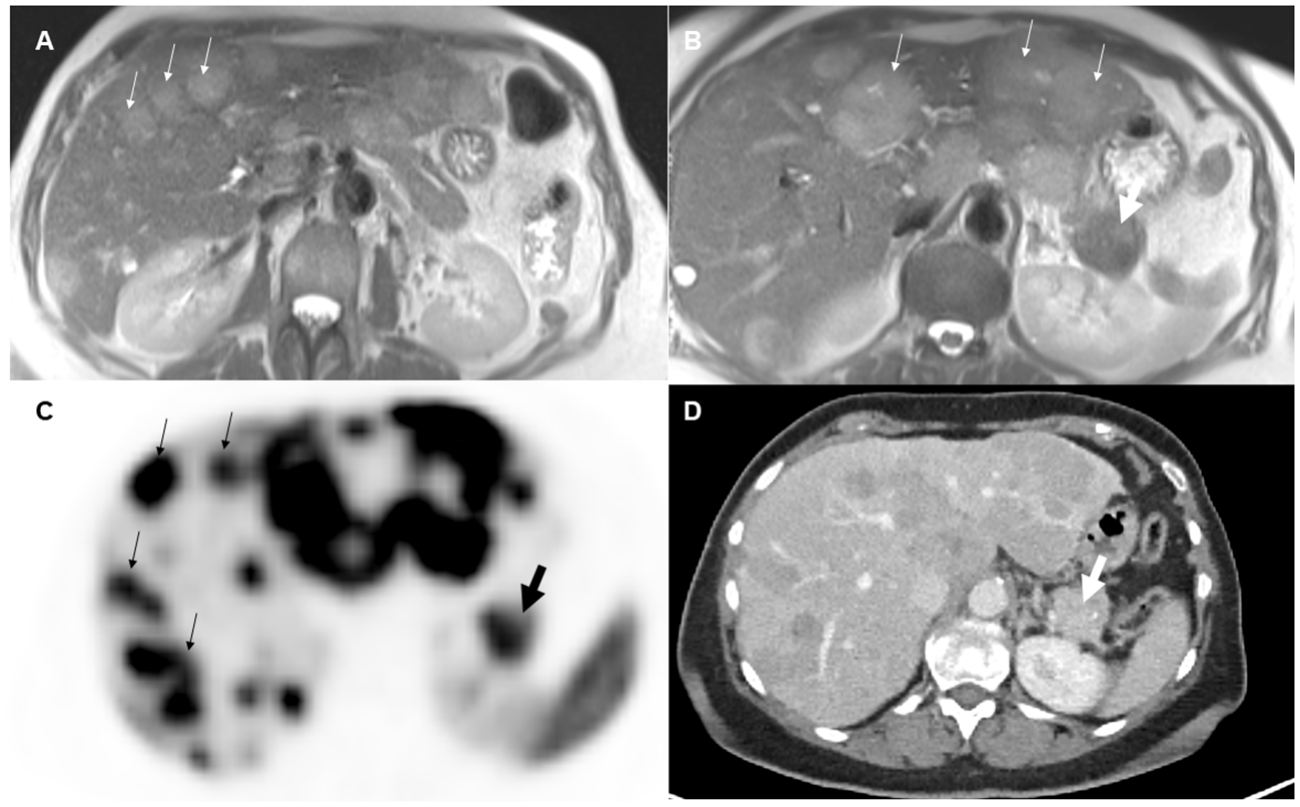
70-year-old female with pancreatic neuroendocrine tumor and synchronous neuroendocrine liver metastases. On T2-weighted images (A, B), the liver metastases (small arrows) appeared significantly more hyperintense than the primary tumor (thick arrow) in the pancreatic tail. On pretherapeutic positron emission tomography-computed tomography examination (C, D), 68Ga-DOTA-TATE tracer uptake was similar between the metastases and the pancreatic neuroendocrine tumor (SUVmean NELM: 35, SUVmean pNET: 32).
